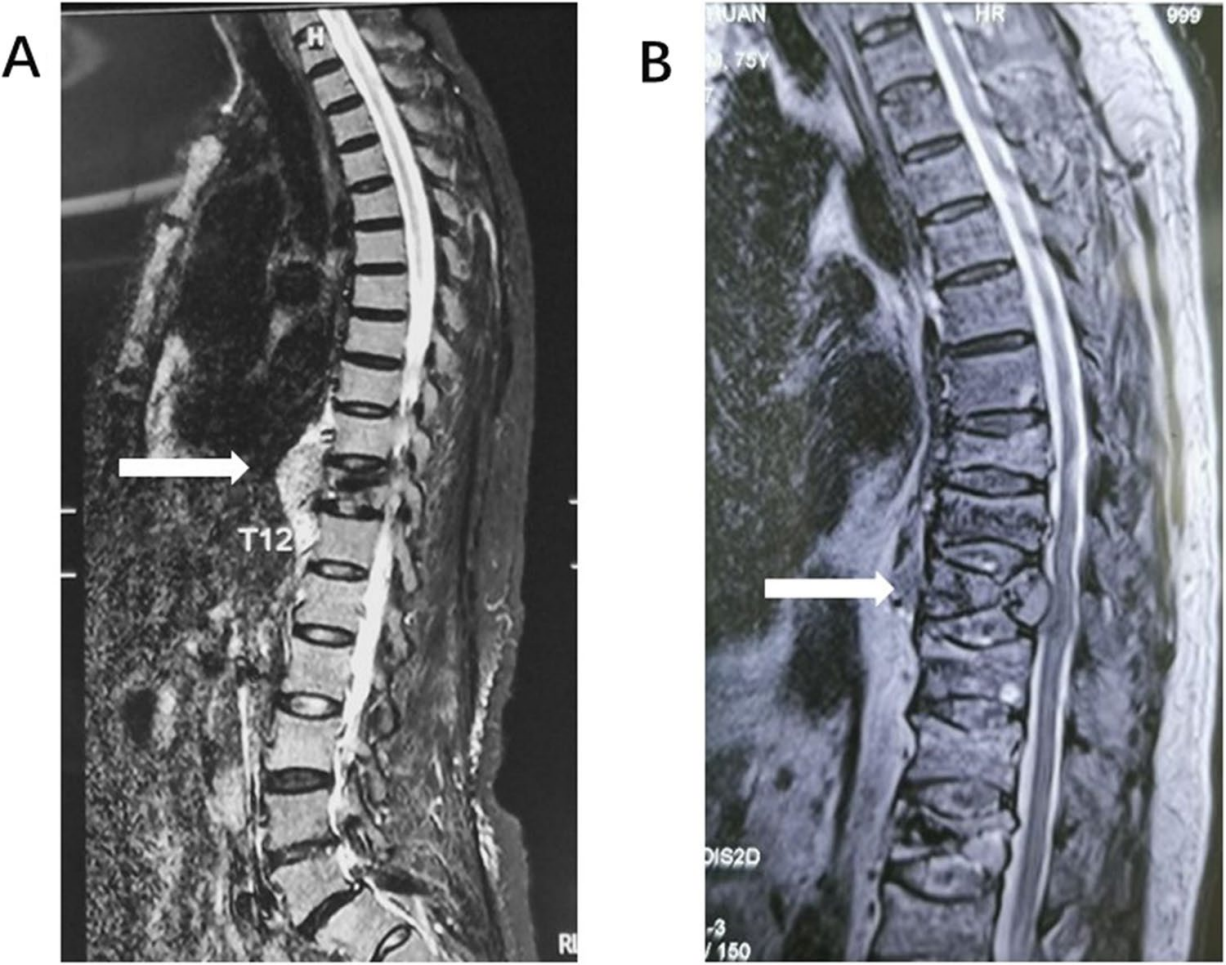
MRI images of case1 and case 2. A MRI image of case 1 shows tumor protrusion into the peritoneum. B MRI image of case 2 shows a T9 compression fracture with a soft tissue mass compressing the spinal cord
